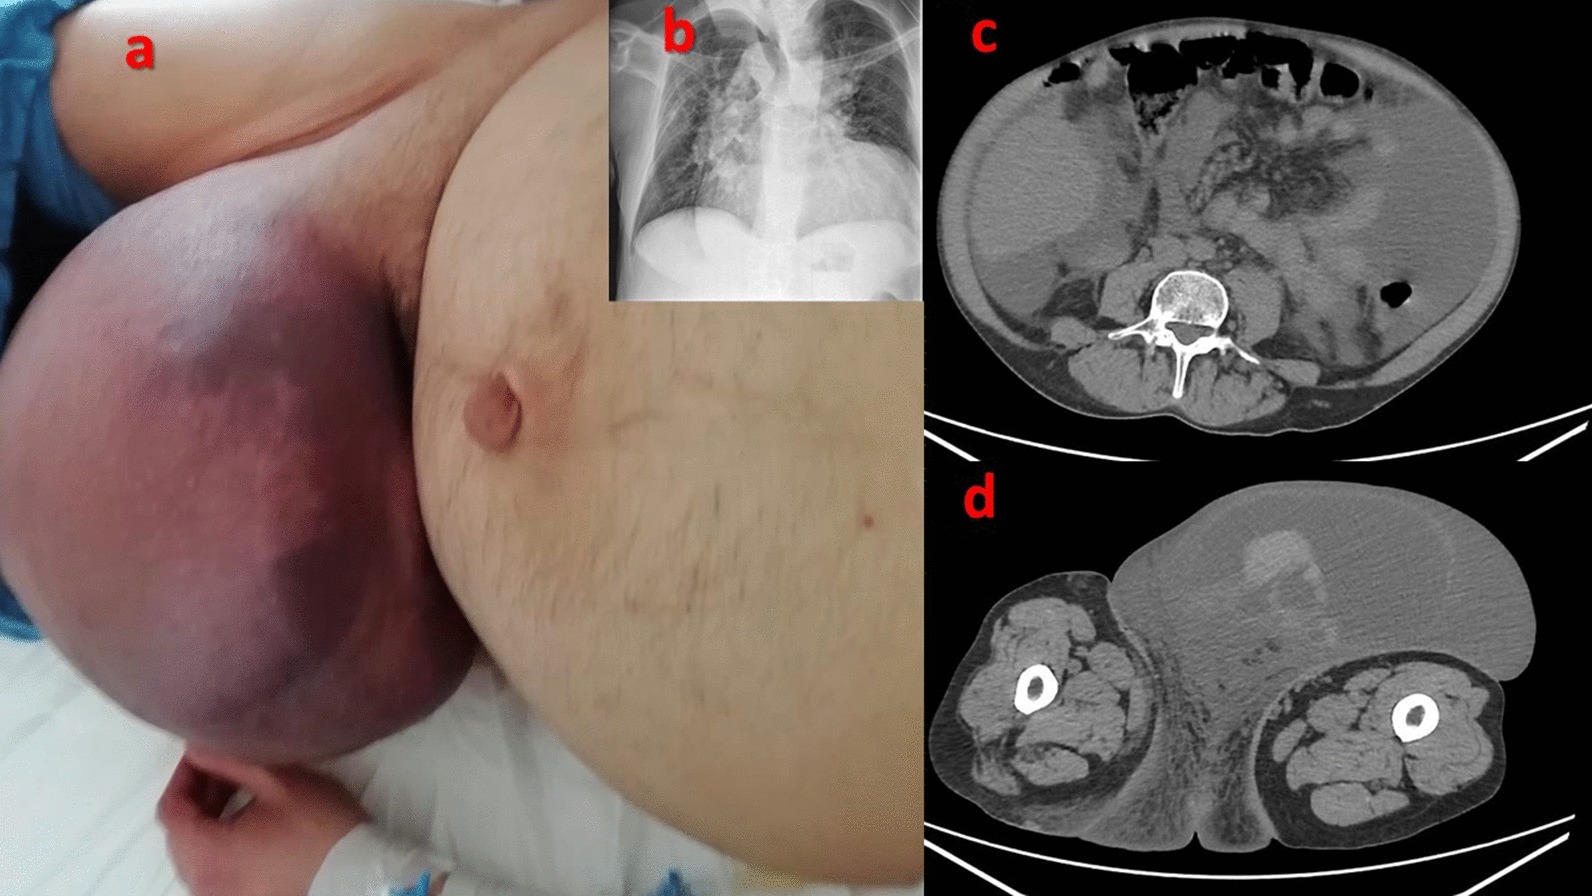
Case 2. a Giant inguino-scrotal hernia apparently strangulated. b Thoracic X-ray showing cardiomegaly (right ventricle hypertrophy). c CT-scan showing copious ascitic fluid in abdomen. d CT-scan ruling out intestinal strangulation
